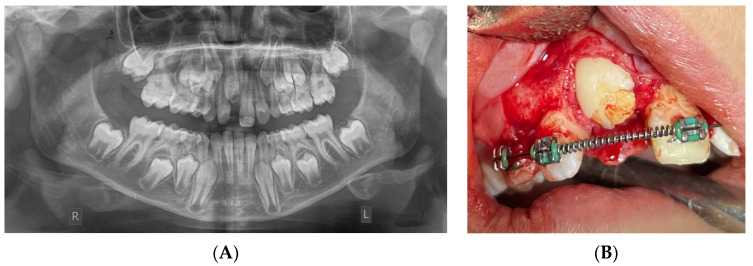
A 9.5-year-old boy presented with impaction of the permanent upper-right central incisor. (A) Panoramic X-ray. (B) Amelogenesis imperfecta was confirmed during the surgical exposure of the impacted tooth.
